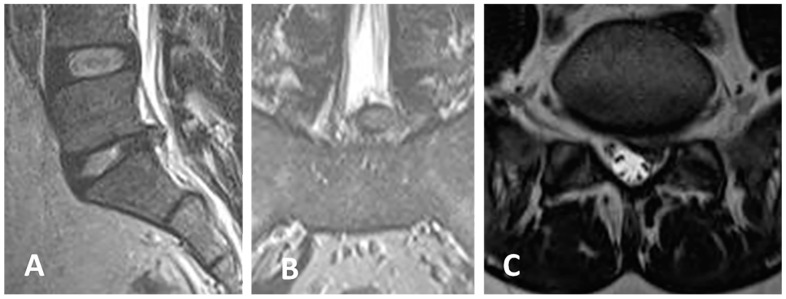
(A–C) Preoperative MRI shows an extruded disc herniation at the left L5-S1 level. VAS was 7, ODI was 60.
